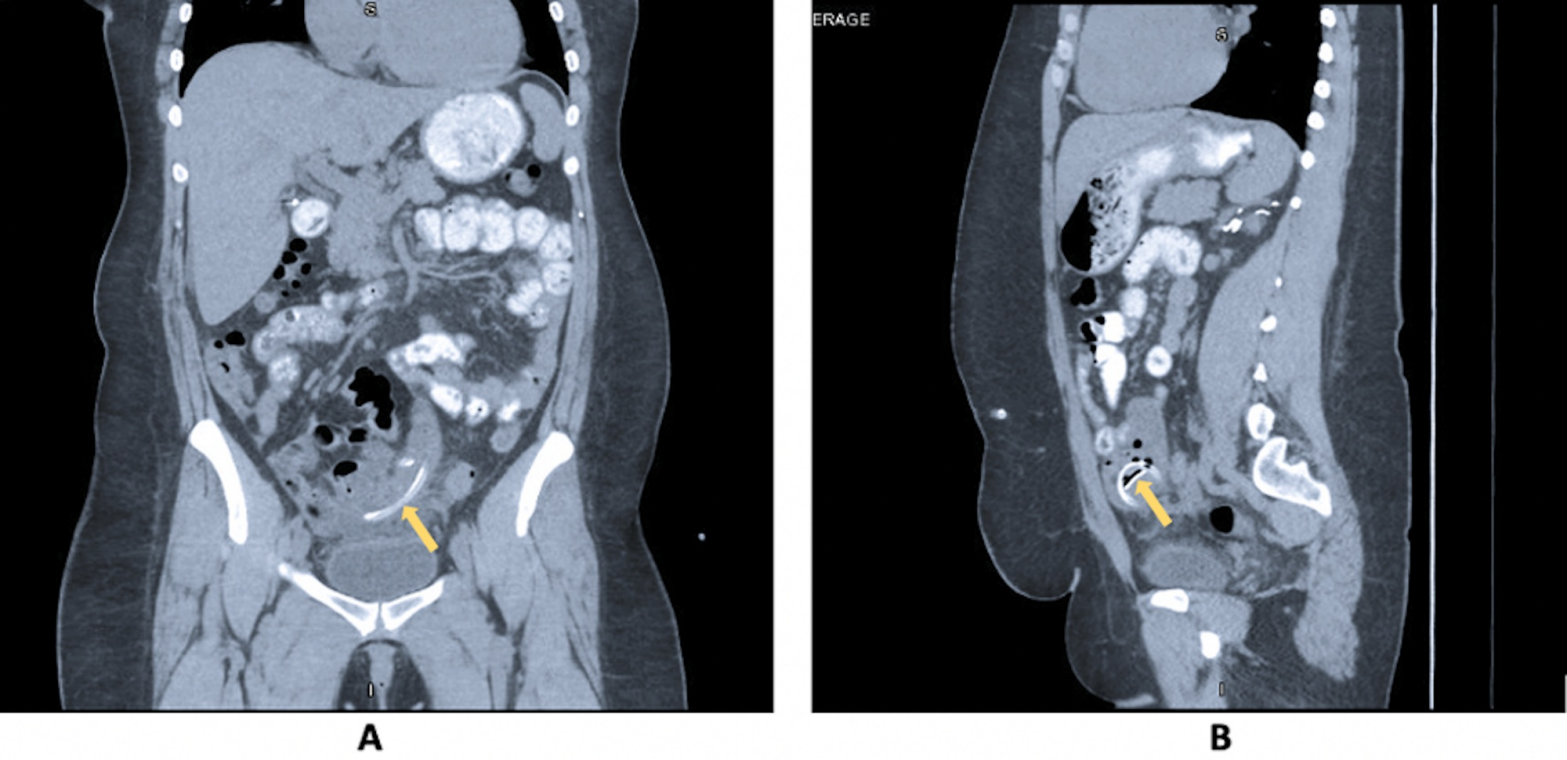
Abdomen/pelvis CT demonstrating peritoneal dialysis catheter appearing to be located within the lumen of the small bowel (yellow arrow, A, B).
